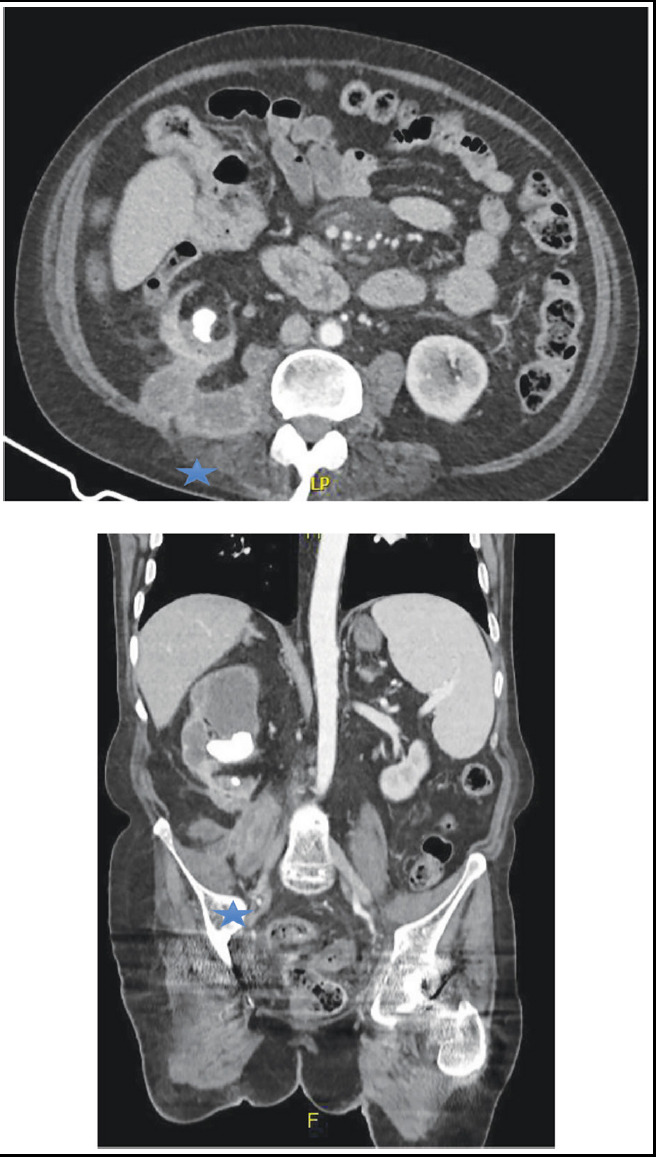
A & B Axial and coronal images of contrast enhanced CT abdomen showing grossly hydronephrotic right kidney with loss pf parenchyma and multiple stones in collecting system. Peri-nephric abscess is also appreciated (Blue star).
